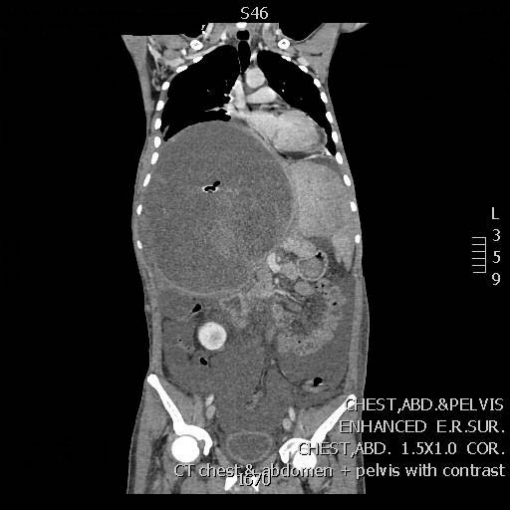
A computed tomography (CT) scan of the abdomen with a large lesion occupying the right hepatic lobe.
